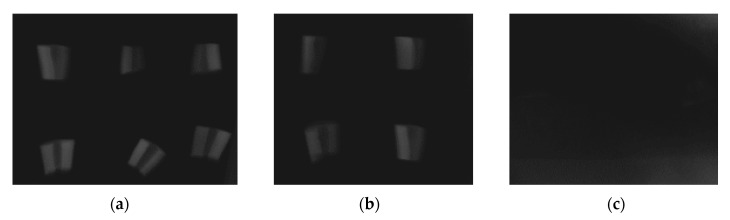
(a) Dental radiographic image of the dentin samples prior to EDTA conditioning. (b) Dentin after 12 h surface decalcification exhibits no visible change in image contrast. (c) After 4 weeks of decalcification, the dentin grafts are no longer discernible in the images.
